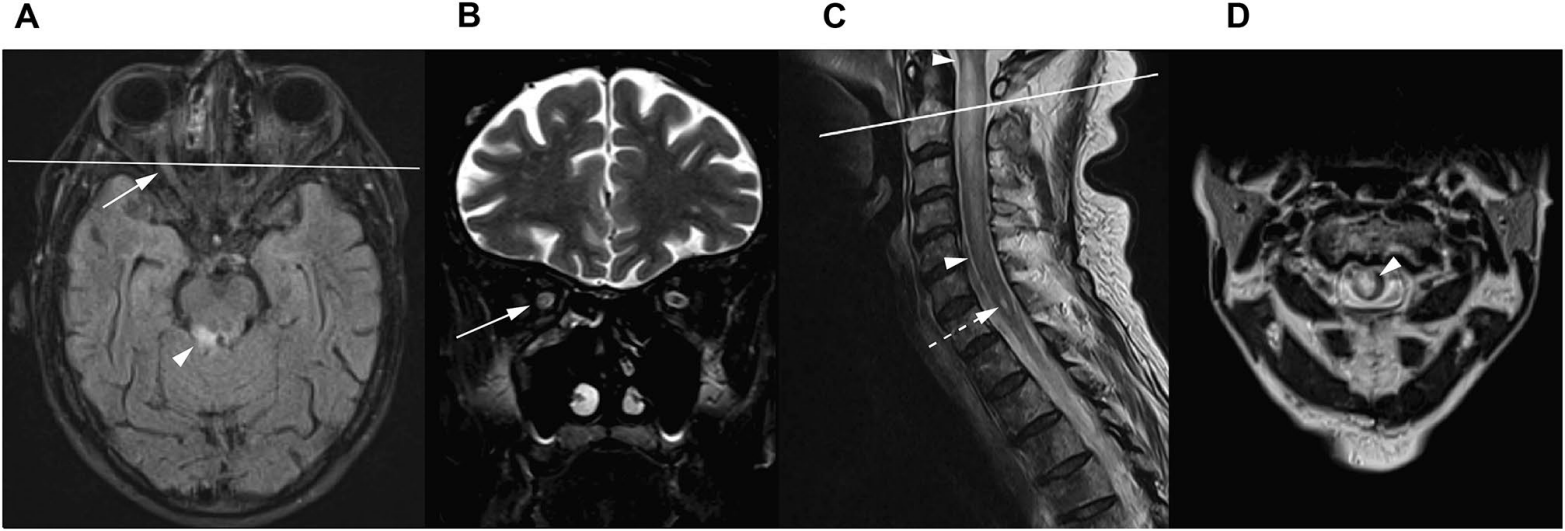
Pathological MRI findings. Axial FLAIR shows periependymal lesions involving the upper section of the pons (arrowhead, A) and upper cerebellar peduncle. Coronal fat-suppressed T2-weighted image shows hyperintense signal of the optic nerve, that is also visible on the axial FLAIR image (arrows, A and B). Sagittal T2-weighted spinal MRI disclosed a longitudinally extensive spinal cord lesion from C1-C5 (arrowheads indicate start and end of the lesion, C and D) and one lateral lesion at C7 (dashed arrow, C). The lines in the first axial and sagittal image indicate the localization of the respective coronar or axial layer.
